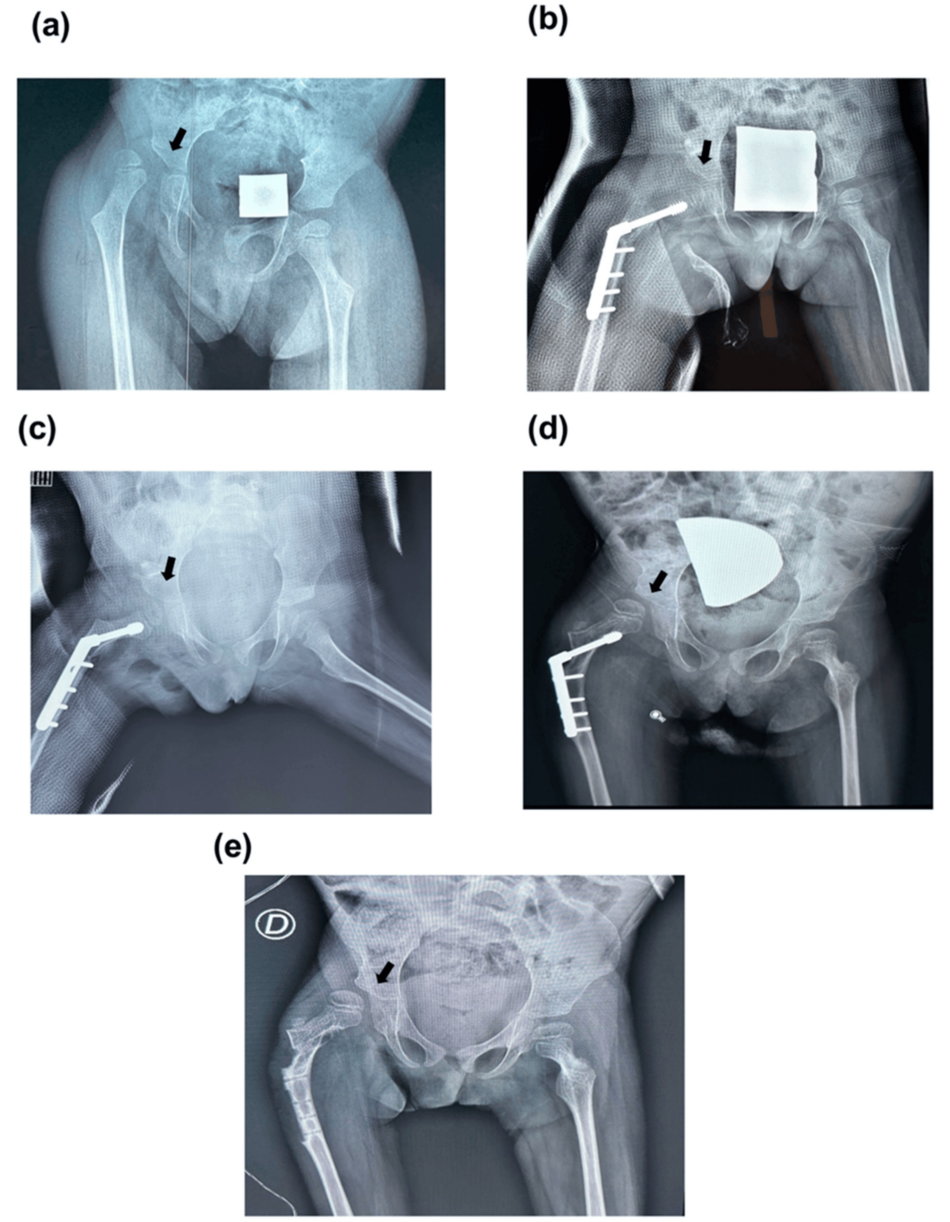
Radiographic representation of a study participant (a) 7- years- old female with spastic quadriplegia and complete dislocation of the right hip. (b) Right hip with VDRO and Pelvic osteotomy. (c) Right hip after six weeks (d). Redislocation of the right hip with an RMP of 50% (e). Right hip with an RMP of 70%. The affected hip is indicated by the black arrow.
